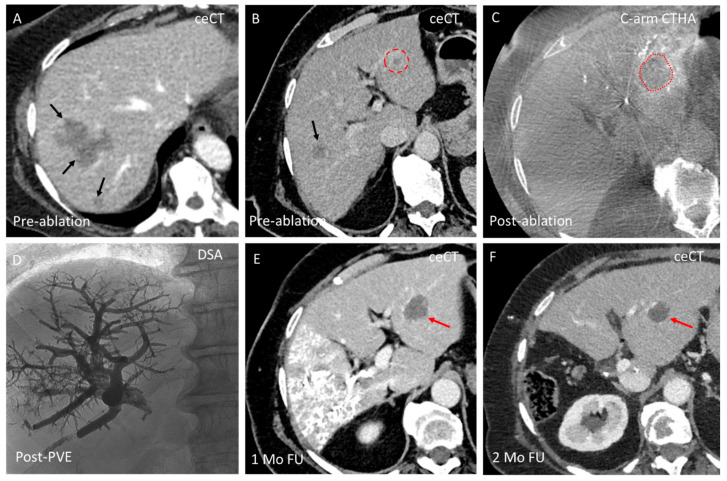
Combined thermal ablation and PVE in a 72-year-old patient with multiple colorectal liver metastases (CRLMs) in the right liver lobe and a 9 mm CRLM in segments 2/3. The patient required a two-stage liver resection: first, inducing future liver remnant (FLR) hypertrophy through PVE of the right portal vein branches, followed by right hemihepatectomy. To prevent progression of the subcentimeter lesion in the FLR, PVE was combined with ablation of this lesion. (A,B) Axial contrast-enhanced CT (ceCT) images, two months before the ablation procedure, show multiple lesions (black arrows) in the right liver lobe and a subcentimeter target lesion in segments 2/3 (red circle); (C) Post-ablation C-arm CT hepatic arteriography (C-arm CTHA) confirms an adequate ablation zone (outlined in red) after 6.5 min at 100 W; (D) Following ablation, the portal vein was percutaneously punctured under ultrasound guidance. A mixture of Glubran 2 and lipiodol (1:8) was injected, successfully occluding all portal vein branches in segments 5 to 8; (E) One-month follow-up (FU) ceCT confirms the desired outcome of the combined procedure: embolization of portal vein branches in segments 5–8 (visible due to the Glubran–lipiodol mixture) has led to FLR hypertrophy. Compared to the pre-ablation ceCT (panel B), the left liver lobe (FLR) has become visibly enlarged. Additionally, the ablation zone (red arrow) in segments 2/3 is visualized, with no signs of residual or recurrent tumor; and (F) Two-month FU ceCT shows the liver remnant, including the ablation zone (red arrow), after successful right hemihepatectomy.
